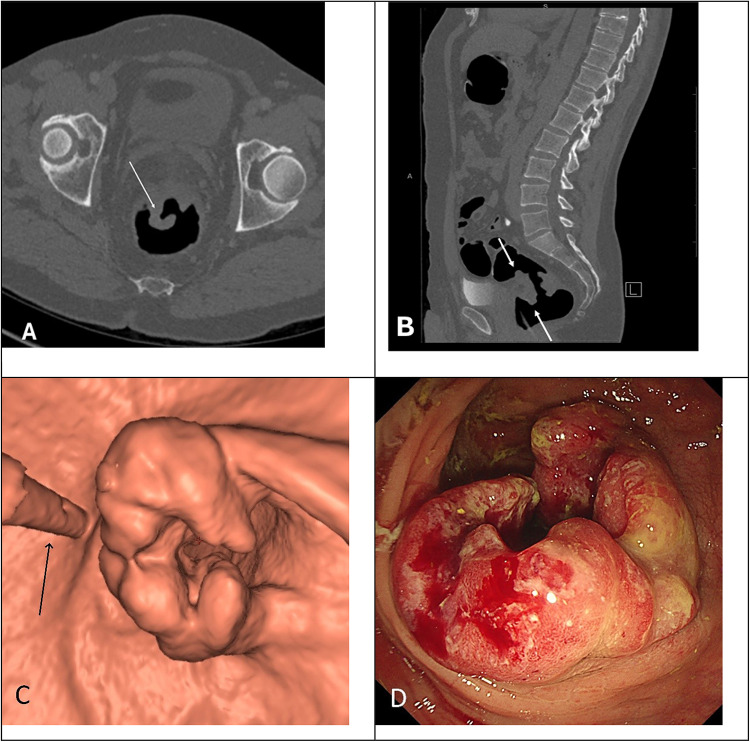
CT colonography examination and endoscopic images of a patient with a locally advanced rectal cancer (subsequently staged by MRI as T4a, N1c, EMVI positive, CRM involved, PSW negative). A shows supine axial view and B the prone sagittal view of the semi-annular lesion in the rectum (solid white arrow), note the rectal catheter (black arrow). Virtual colonoscopic image is shown in C (note the rectal catheter, black arrow) and the endoscopic image in D (note the different orientation between endoscopic and CT images). *EMVI* extramural vascular invasion, *CRM* circumferential resection margin, *PSW* pelvic side wall lymph node
